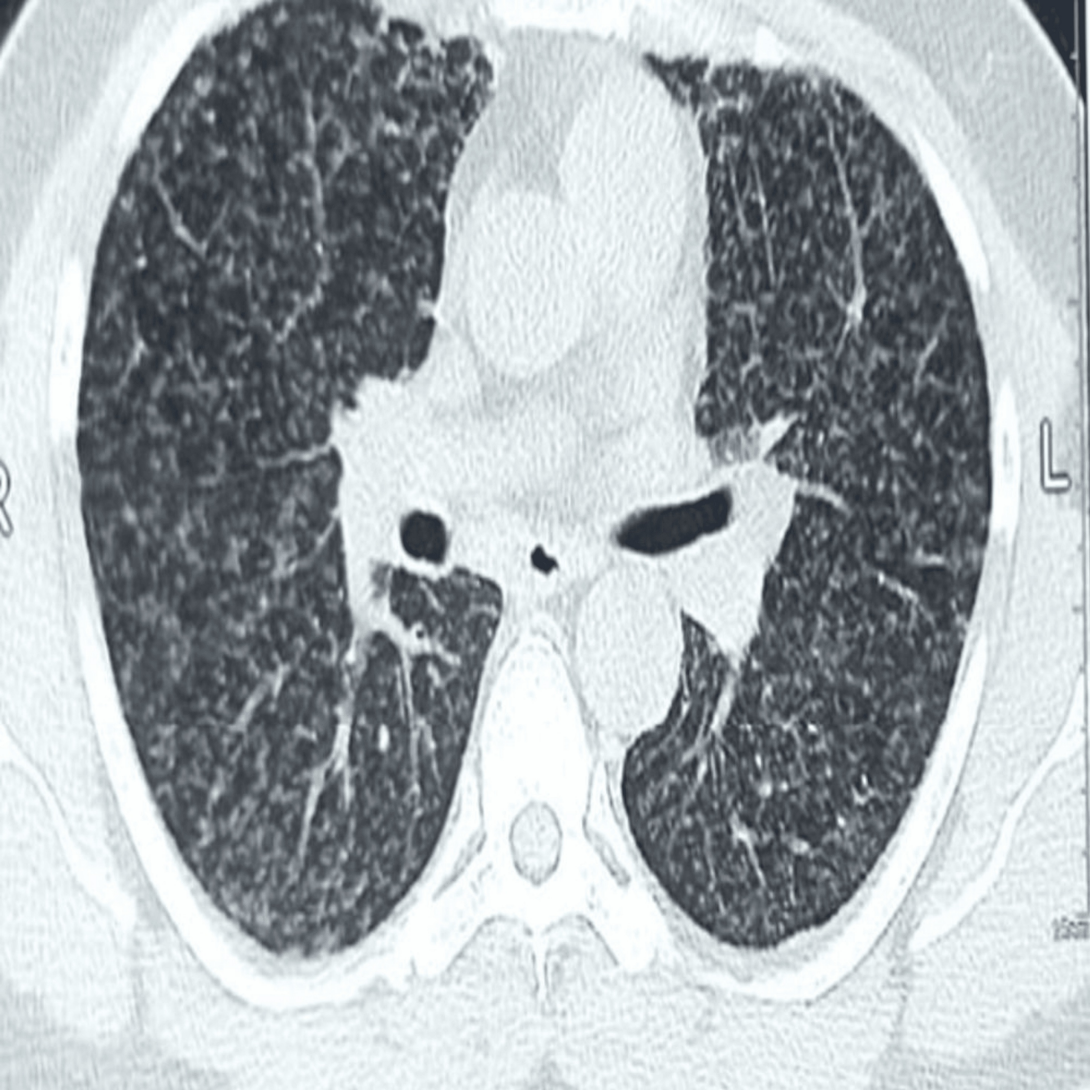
Transverse computed tomography of the lung window reveals a miliary nodular opacity all over the lung field
